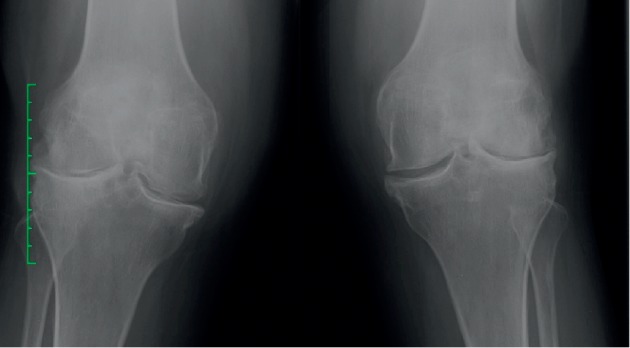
X-ray examination in case 3.
